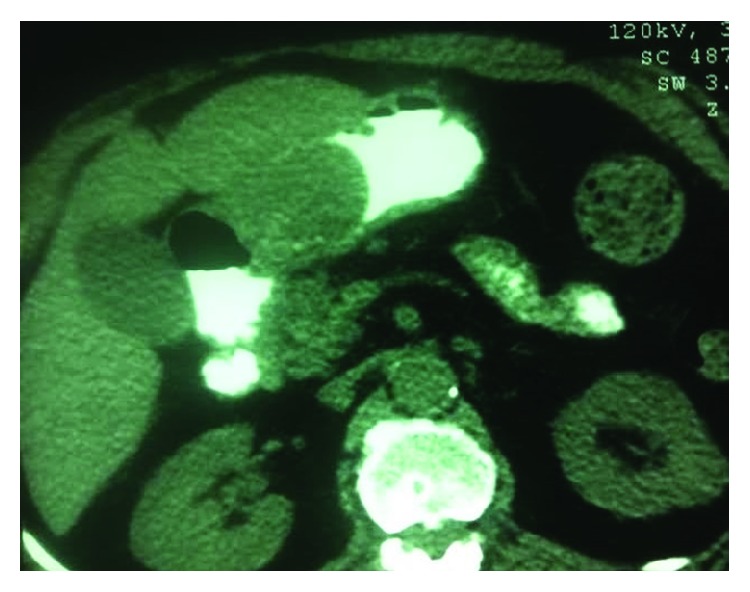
Computed tomography scan of the abdomen demonstrating a well-defined soft tissue mass arising from the antrum.
